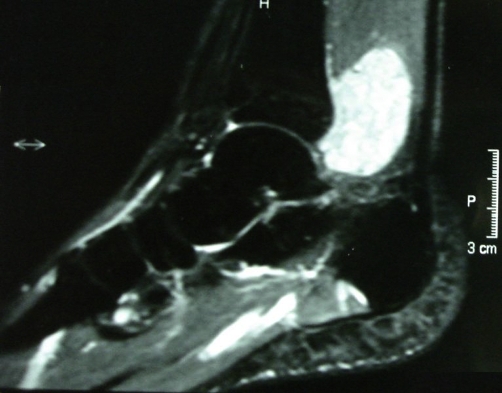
Preoperative T2-weighted MR image demonstrates a high-intensity heterogenous mass in the same place.
